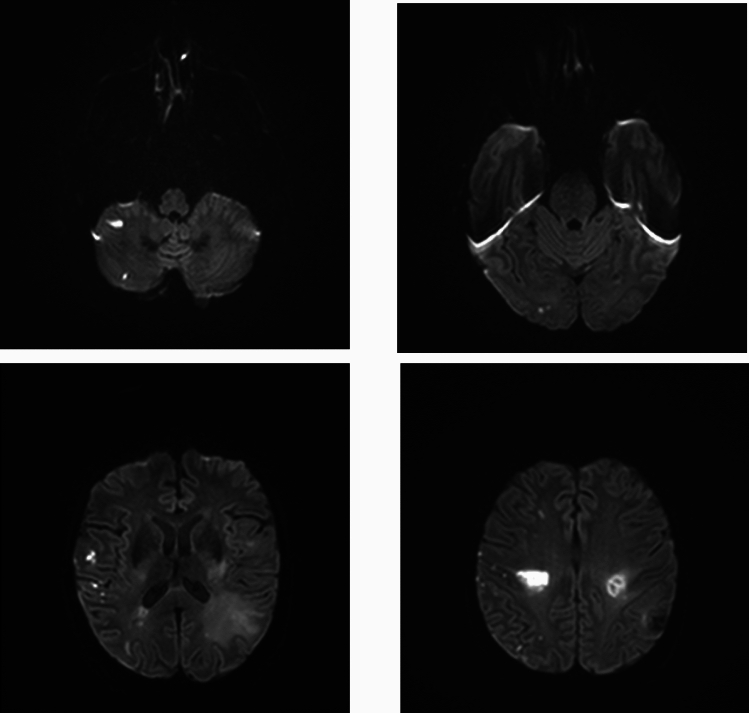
Axial diffusion-weighted MRI demonstrating multiple, metachronous ischemic infarctions in bilateral middle cerebral artery territories and in the posterior circulation (“three-territory sign”) in a patient with active malignancy and non-bacterial thrombotic endocarditis. Abbreviations: MRI, magnetic resonance imaging.
